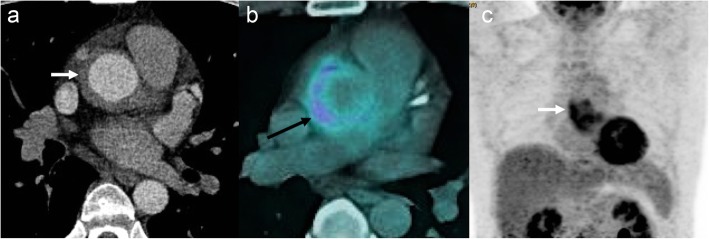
A 47-year-old male with atypical chest pain. Axial CTA image (a) shows circumferential wall thickening (arrow) of the ascending aorta. Axial fused and coronal maximum intensity projection (MIP) F-18 FDG PET-CT images (b and c) shows increased FDG uptake in aortic wall (arrow) consistent with active vasculitis (temporal artery biopsy showed giant cell arteritis)
